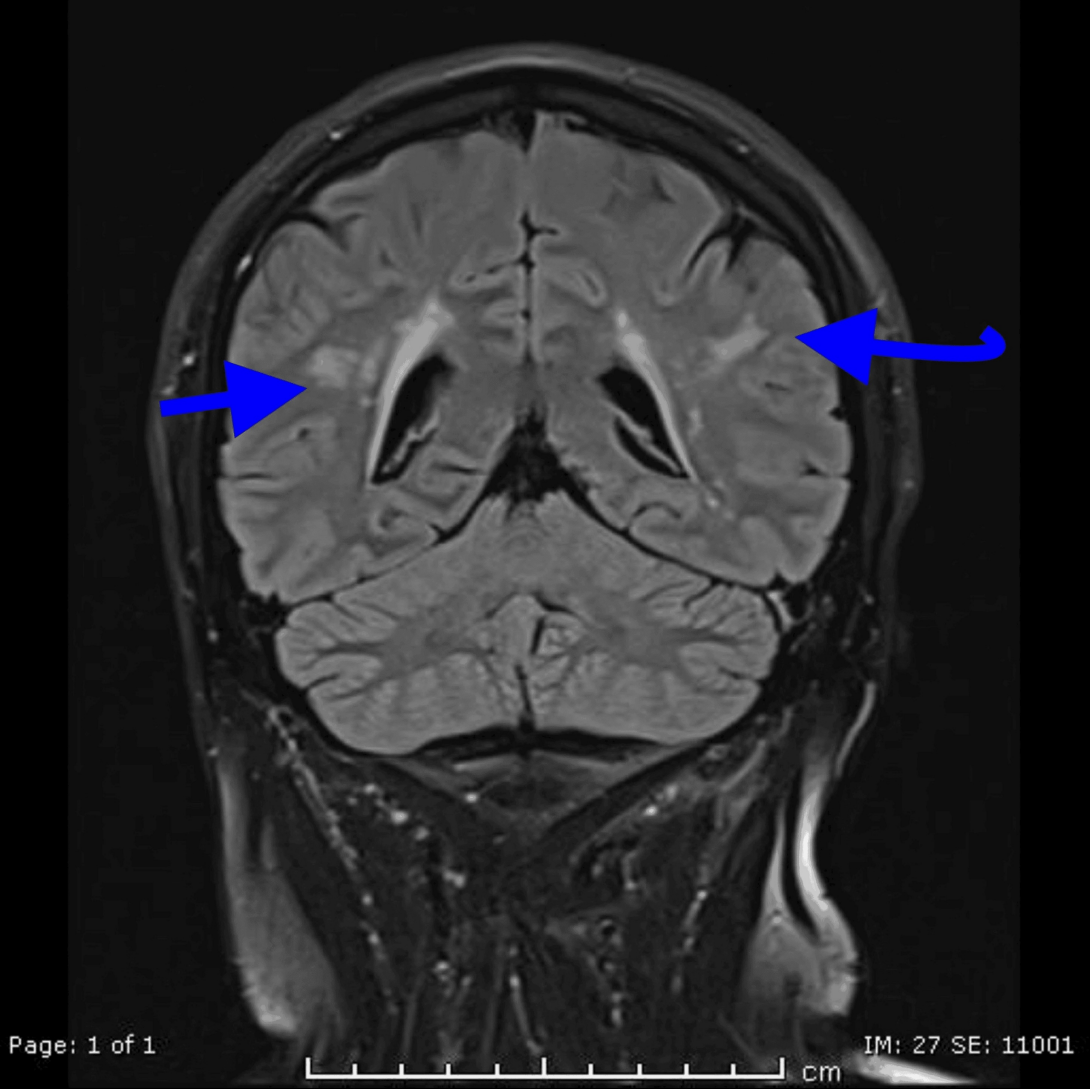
MRI T2 coronal view of the patient shows the presence of a high T2 signal in the subcortical white matter of the frontal and parietal lobes, which is a characteristic radiological feature of CADASIL CADASIL, cerebral autosomal dominant arteriopathy with subcortical infarcts and leukoencephalopathy
